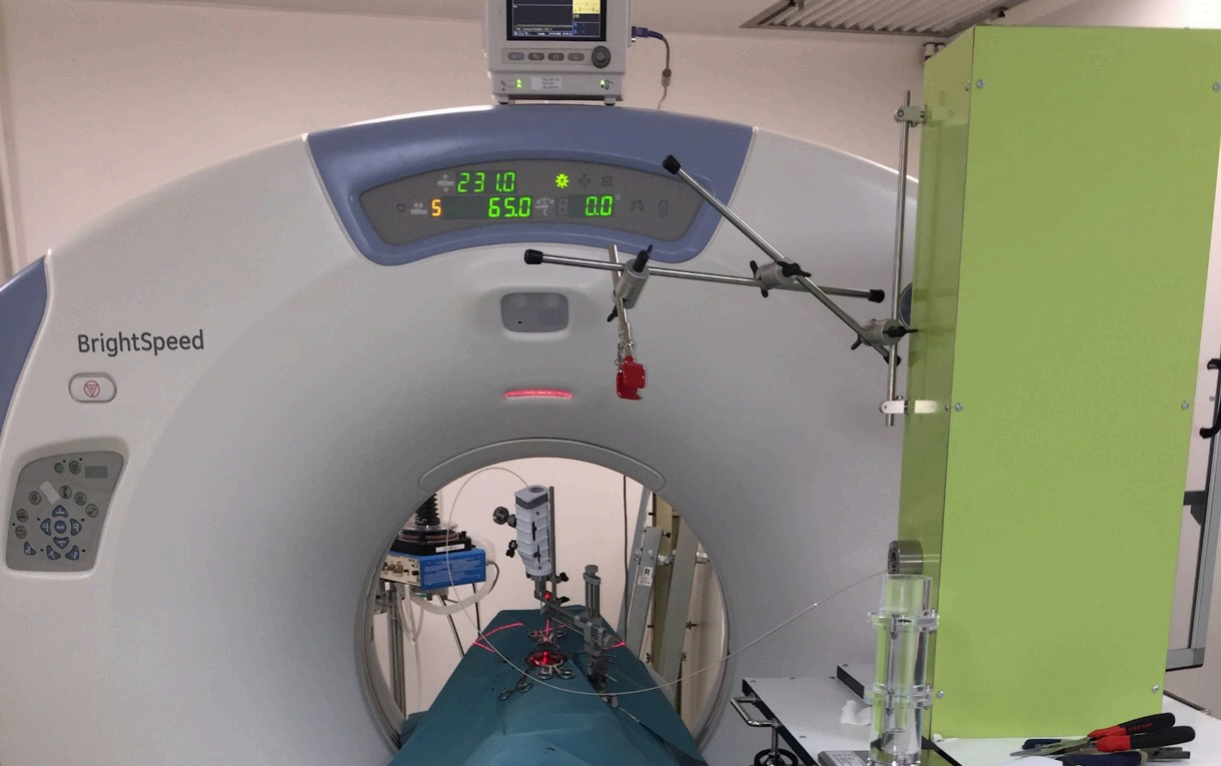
Preclinical injection system developed by EVEON. The injector is located beside the operation table in CT room. The injection needle is connected to the injector through a connection tube to transfer the Ho suspension from the injector to the needle.
